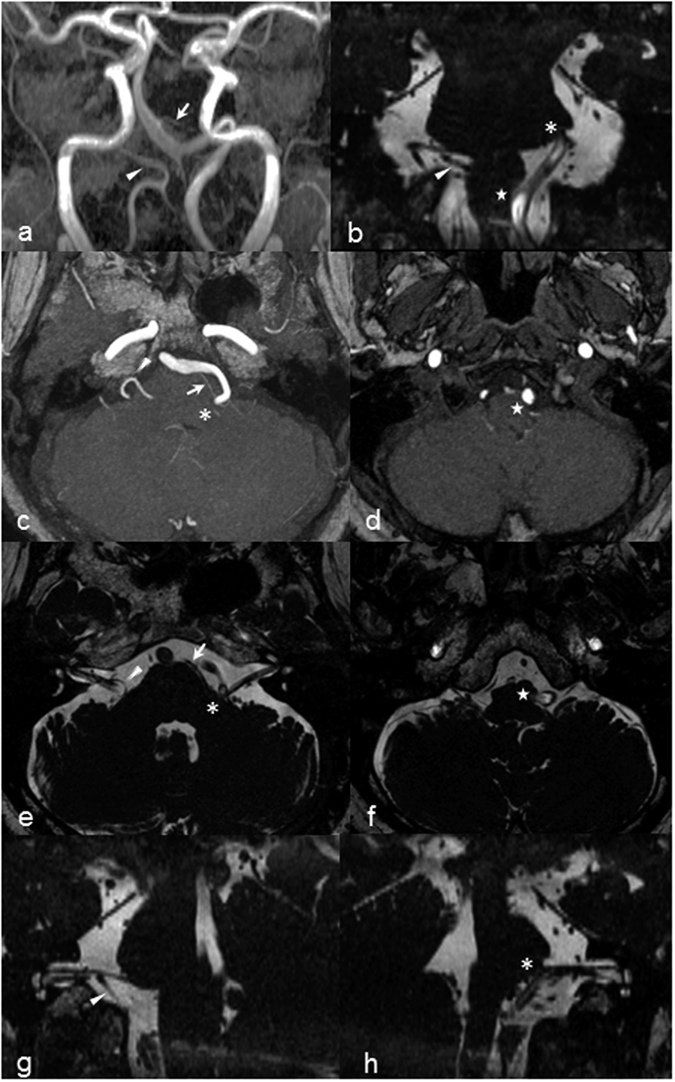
Brain MRI of a hypertensive male with left hemifacial spasm. (a) Coronal MRA MIP image showing dolichoectatic of the vertebrobasilar arteries. (b) Coronal CISS image showing compression and indentation on the left facial nerve REZ and left VLM by the tortuous left VA. Axial MRA MIP images showing vascular compression at the level of the (c) pons by the left VA and AICA, and (d) medulla by the left VA. Corresponding axial CISS images detailing compression at the left (e) facial nerve REZ and (f) VLM. Oblique sagittal reconstructed CISS images along the facial nerve on the (g) right showing normal lie of the nerve with PICA nearby and pregnant pontine belly, and (h) left showing pontine distortion and elevated facial nerve REZ. (Abbreviations: AICA = anterior inferior cerebellar artery; CISS = constructive interference at steady state; MIP = maximum intensity projection; MRA = magnetic resonance angiography; PICA = posterior inferior cerebellar artery; REZ = root exit zone; vertebral artery = VA; VLM = ventrolateral medulla. Annotations: arrow = left AICA ; arrowhead = right PICA, asterisk = REZ of left facial nerve; star = left VLM compression).
